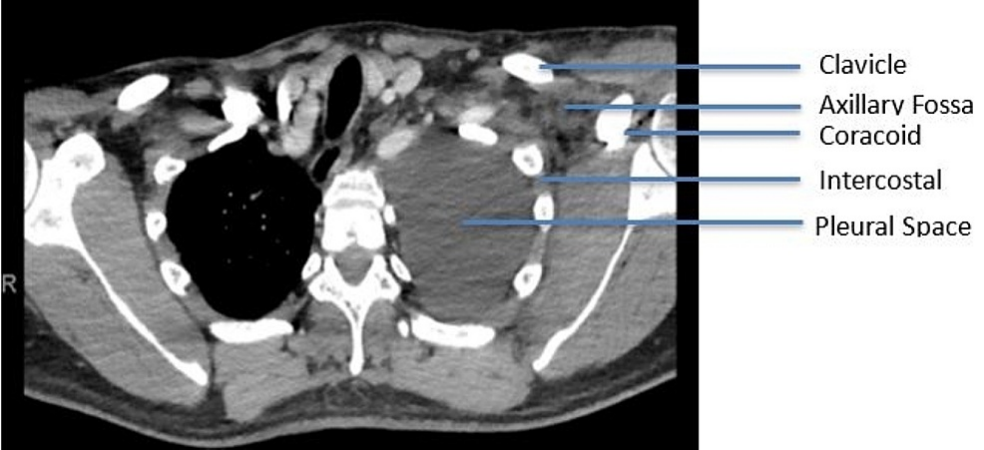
Postoperative axial slice from the CT chest revealing anatomic relationship of surgical site to pleural space Layers include coracoid process, axillary fossa, intercostal muscles, parietal pleura, and pleural space.
